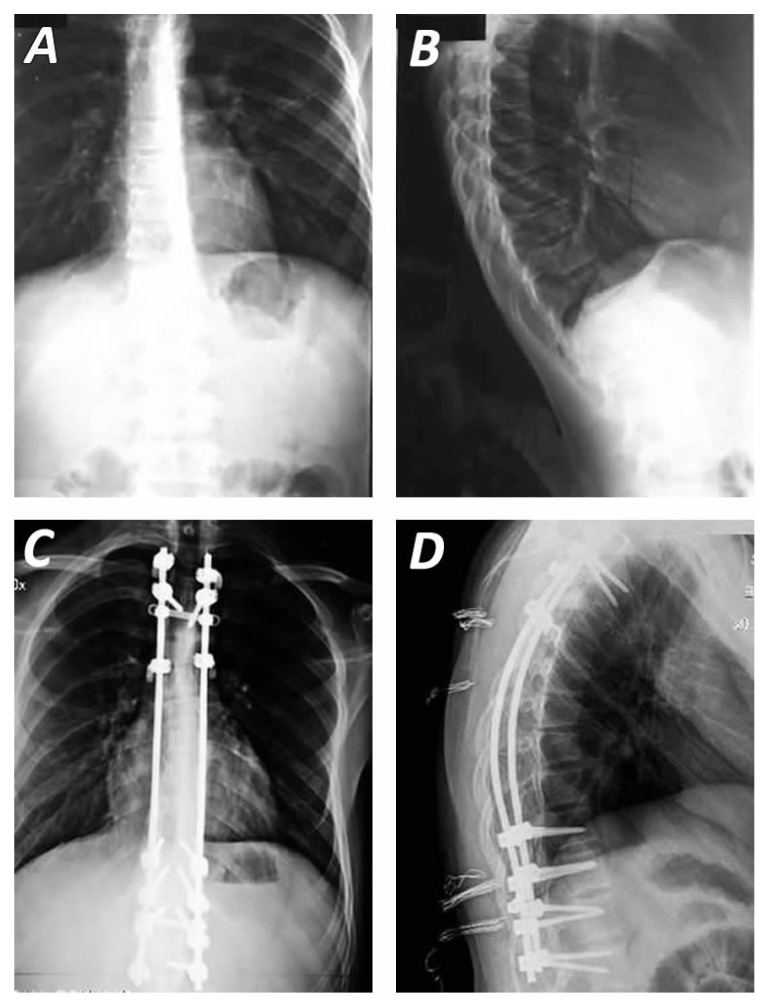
Pre-(A,B) and postoperative (C,D) radiographs of an 18-year-old male patient with Scheuermann’s kyphosis, depicting thoracic kyphosis and the correction of deformity. Posterior spinal fusion (T3–L2) was performed with use of pedicle screws (T4, T11, T12, L1, L2) and transverse process hooks (T3, T6) connected to dual pre-curved compression rods accompanied with two cross-links. Facet osteotomies (T6, T7, T8, T9, T10) and spinous process removal (T6, T7, T8, T9, T10) were necessary for curvature correction and fusion along with use of allograft (C,D). The preoperative kyphosis was 79° degrees extended from T3 to L2 (B), while after the fusion of T3 to L2 the postoperative angle was 38–40° degrees (D).
